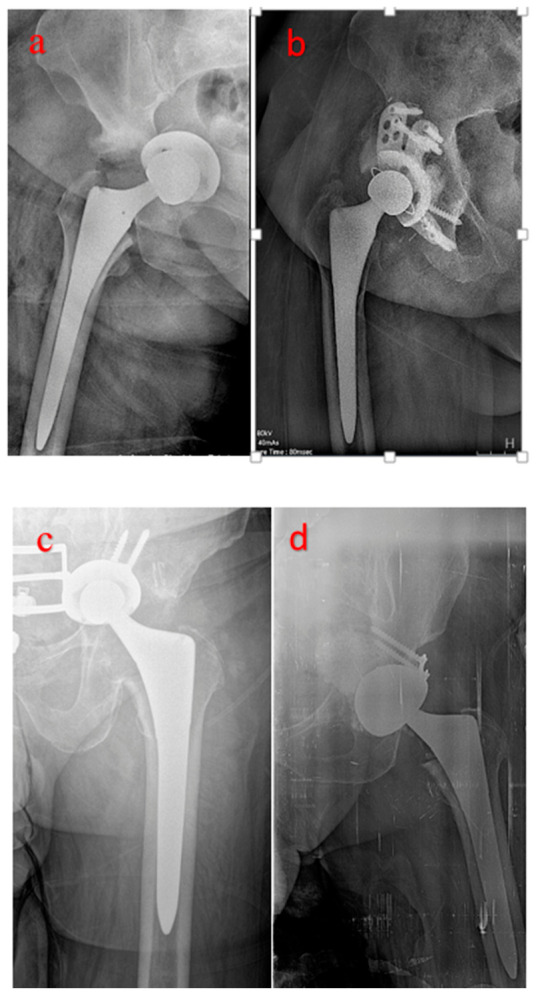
(a) Preoperative radiograph showing a Paprosky Type IIC acetabular defect; (b) postoperative view following cage reconstruction. (c) Preoperative radiograph showing a Paprosky Type IIC acetabular defect; (d) postoperative view following impaction bone grafting + dual mobility cup reconstruction. Preoperative radiographs demonstrate characteristic features of Paprosky Type IIC defects, including medial wall deficiency (Kohler’s line disruption), medial migration of the hip center, and preservation of the superior dome. Given the potential for overlap with more advanced defect patterns, some degree of misclassification cannot be excluded.
